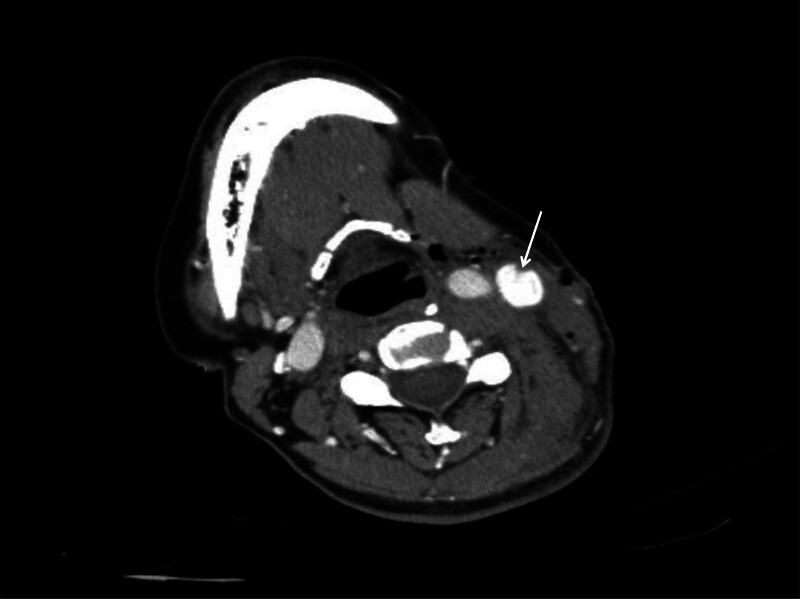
Neck-enhanced CT. The arrow indicates the injury at the left internal jugular vein. CT = computed tomography.
